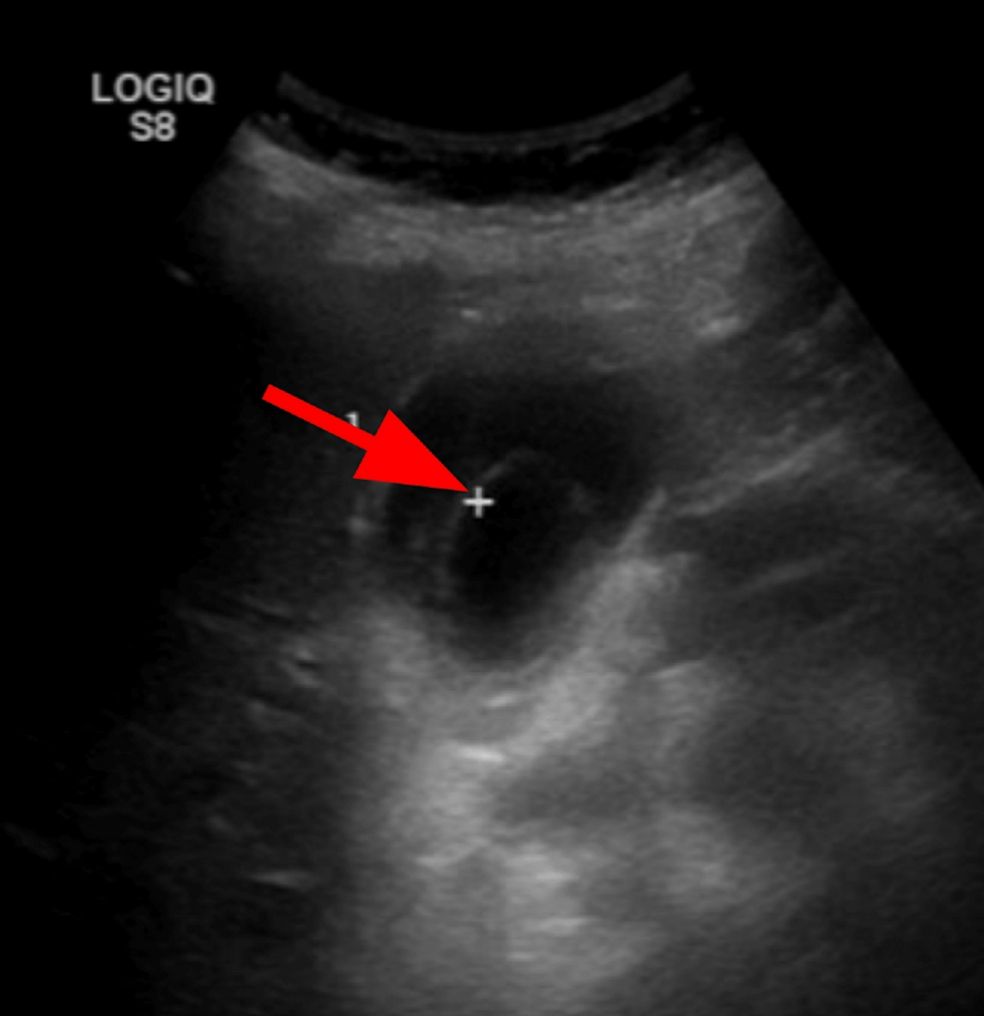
Right upper quadrant ultrasound Ultrasound showing delimitation of the gallbladder lumen, findings consistent with sloughed mucosa (solid arrow) seen in membranous gangrenous cholecystitis.
